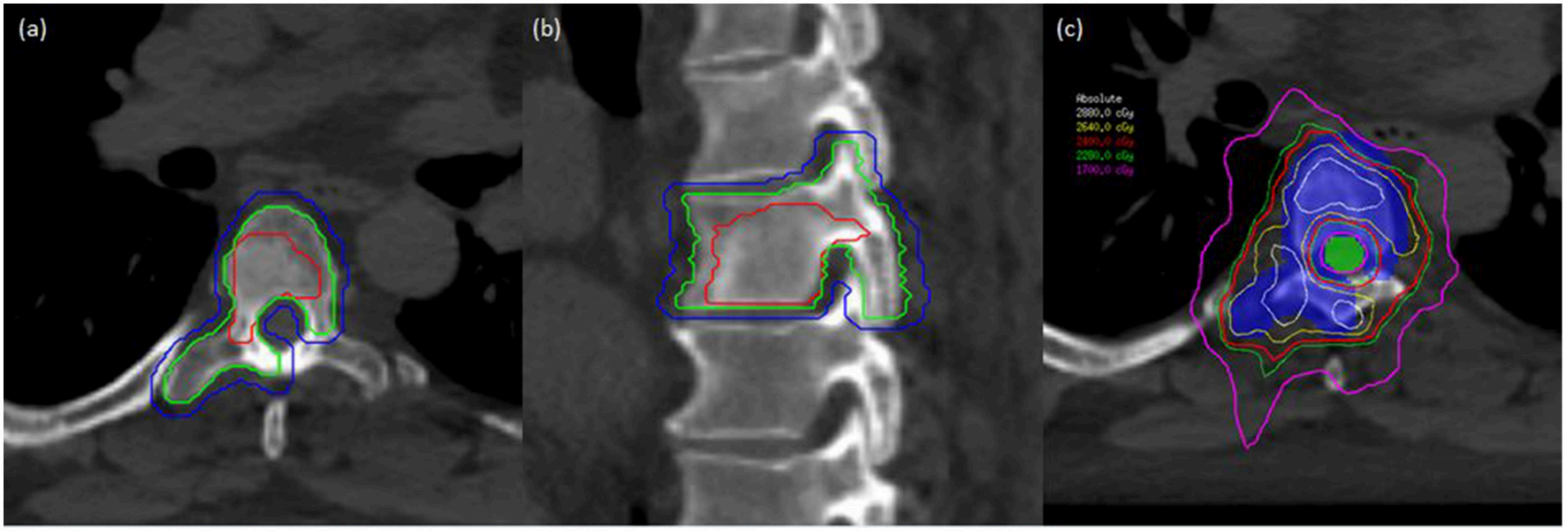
A man with oligometastatic castrate-resistant prostate cancer with painful spine metastases. This man was treated to 24 Gy in 2 fractions. (a) Axial planning CT scan demonstrating T6 vertebral level with gross tumor volume (GTV), clinical target volume (CTV), and planning target volume (PTV) delineated with red, green, and blue lines, respectively. (b) Sagittal planning CT demonstrating T6 vertebral level with GTV, CTV, and PTV in red, green, and blue, respectively. (c) Dose distribution at the level of T6 with PTV (colorwash blue) and spinal cord planning organ at risk volume (PRV) in colorwash green. Demonstration of sharp-dose fall-off to respect critical structures while allowing coverage of the target volumes.
